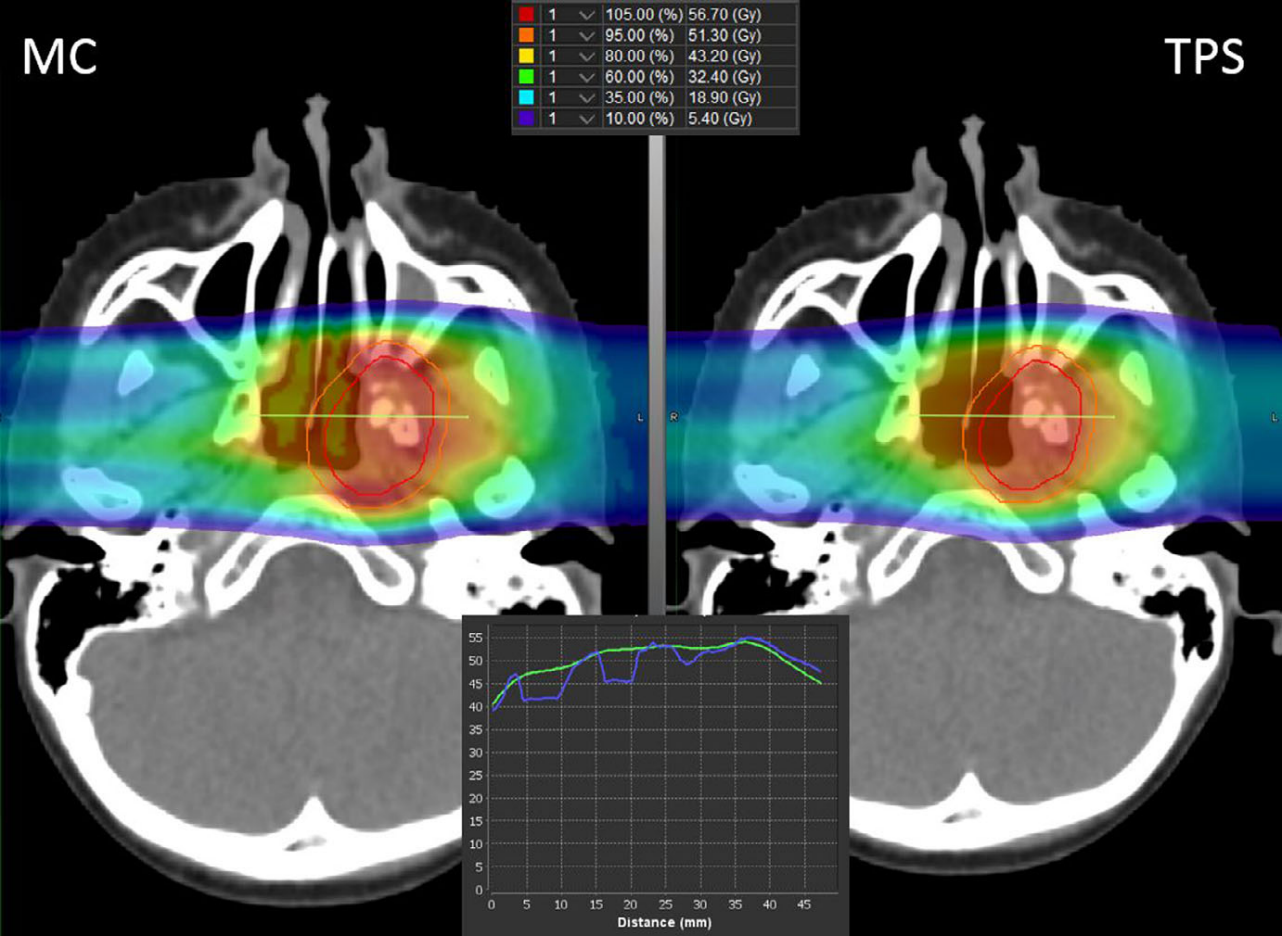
Dose Comparison of treatment planning systems (TPS) and MC – Axial slice of a patient's CT, with MC (left) and TPS (right) dose shown as a color wash. The red and orange contours represent gross tumor volume and clinical target volume respectively. An inset of a dose profile through the target volumes shows the TPS dose (green) and MC dose (blue)
